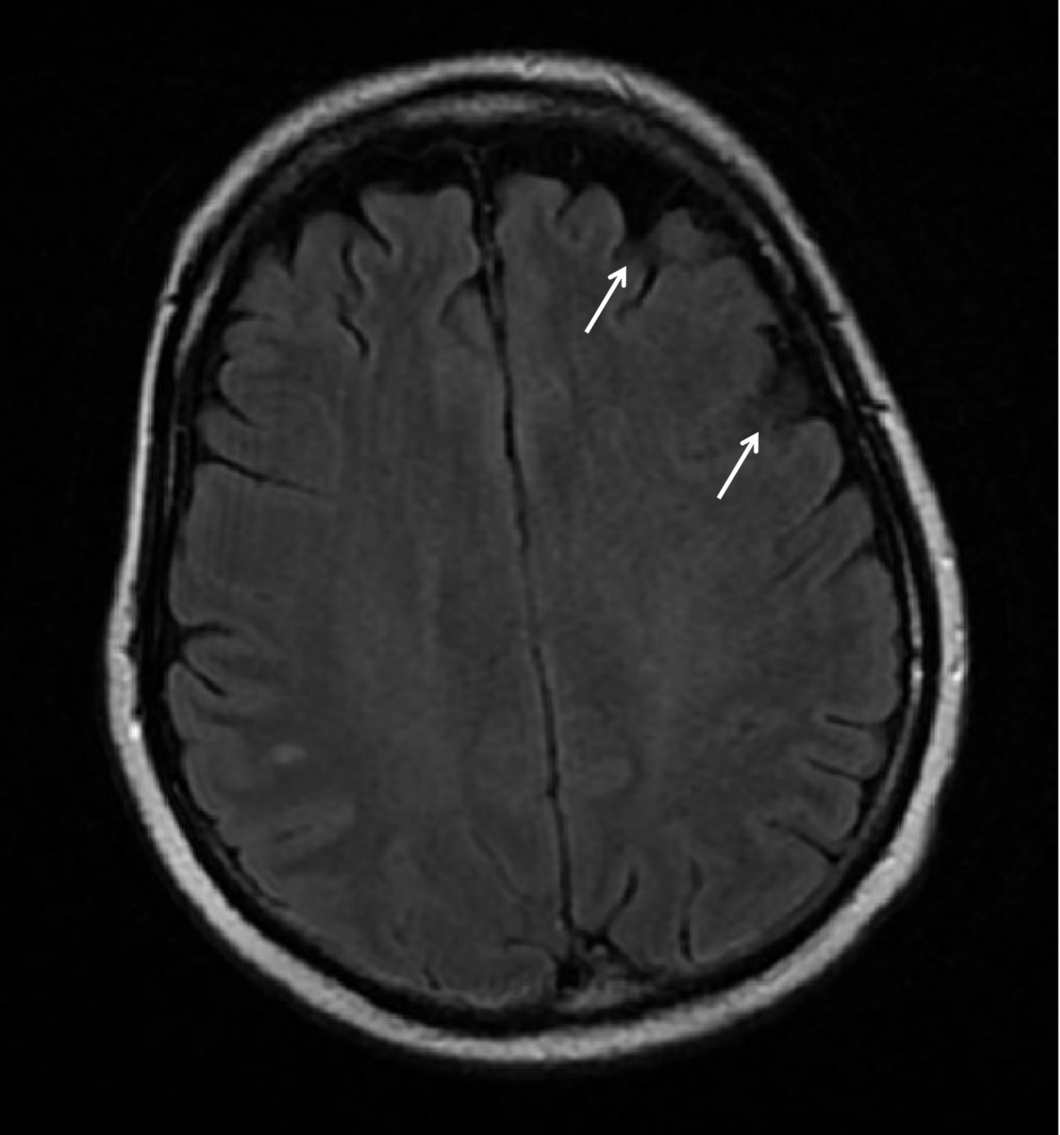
Axial MRI of the brain showing hypointense left frontoparietal lesions (white arrows) without midline shift
